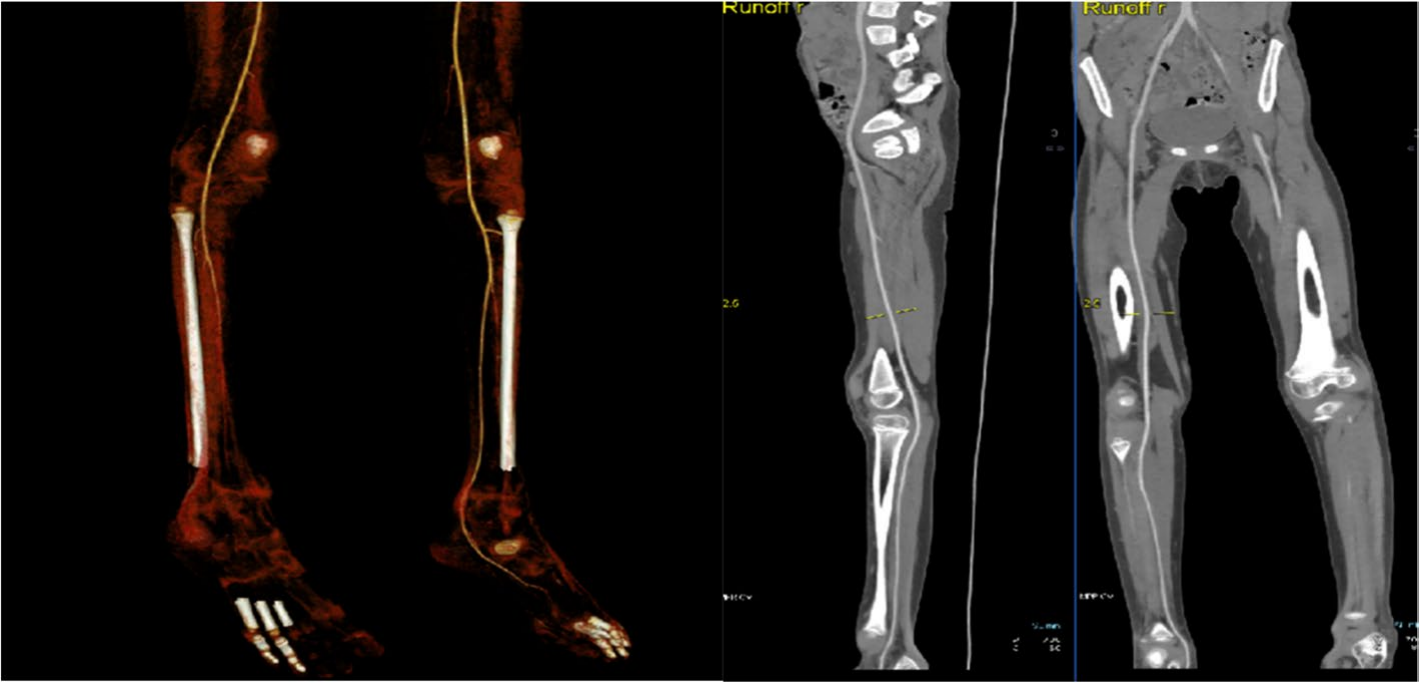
Computed tomography angiogram of the lower limbs showed a mild to moderate attenuation of the right anterior and posterior tibial and dorsalis pedis
